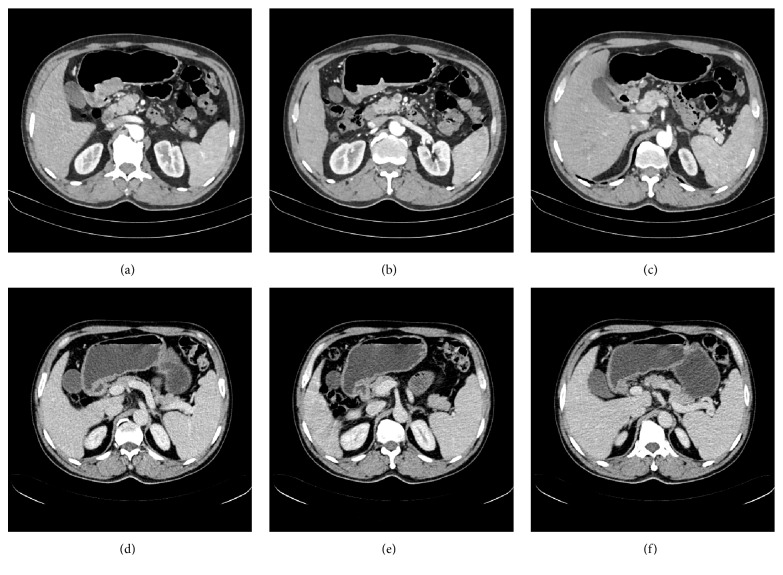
Initial abdominal computed tomography scan (a~c) and follow-up scan after three cycles of EOX chemotherapy (d~f). (a, b, and c) Irregular wall thickening on the lesser curvature side of the gastric antrum with mass formation, large ulceroinfiltrative, and multiple metastases to neighboring lymph nodes; (d, e) scan shows that the mass of the gastric antrum decreased in range from approximately 7 cm to less than 3.3 cm; (f) disappearance of metastatic lesion of neighboring lymph nodes that had been previously observed.
